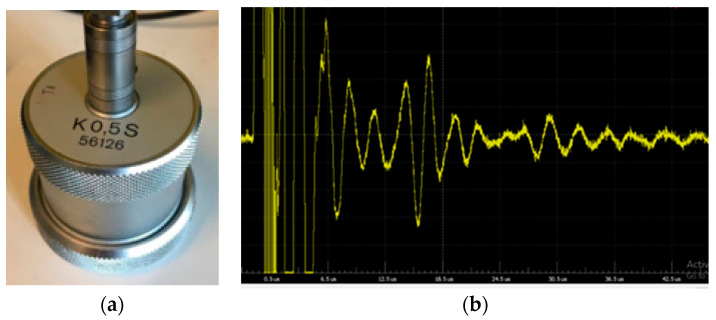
(a) An ultrasound sensor; (b) A-Scan from an ultrasound sensor.
